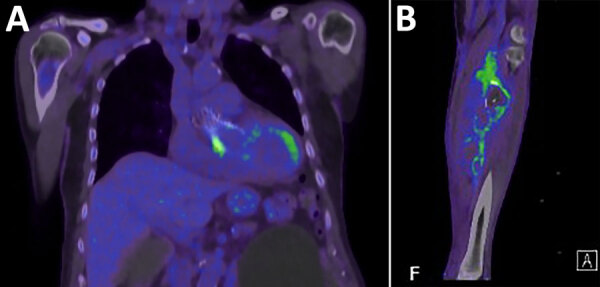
Positron emission tomography/computed tomography images from study of patient with *Bartonella clarridgeiae* prosthetic-valve endocarditis and aortic root abscess, Australia, 2020. A) Fluorodeoxyglucose activity at ventricular end of the transcatheter aortic valve implantation; B) repaired tibioperoneal trunk aneurysm in patient’s left leg.
